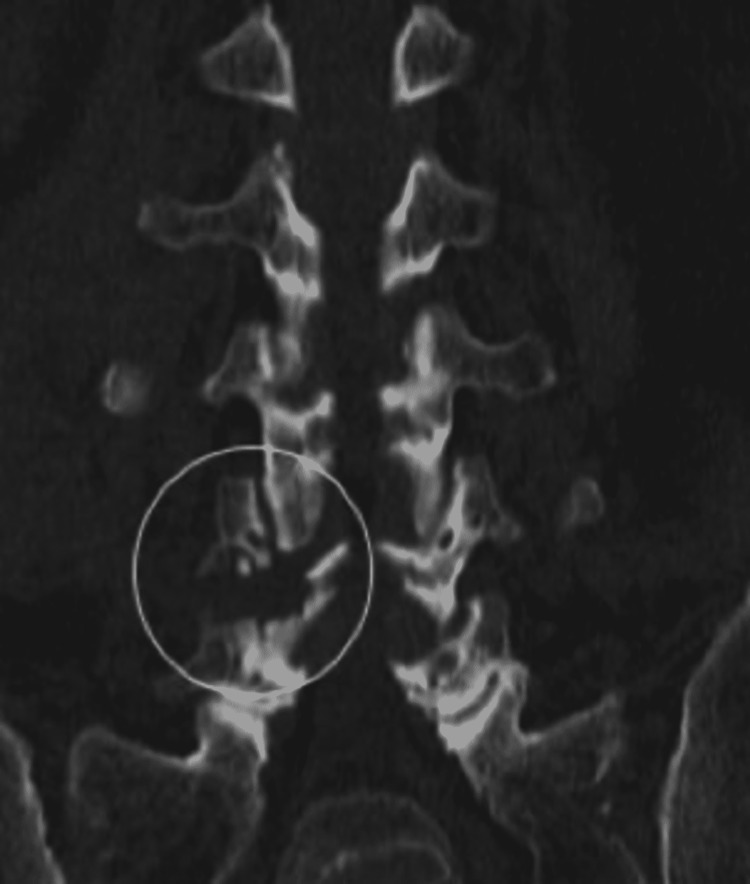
Postoperative lumbar coronal CT shows partial resection of the pars inter articulation (white circle)
